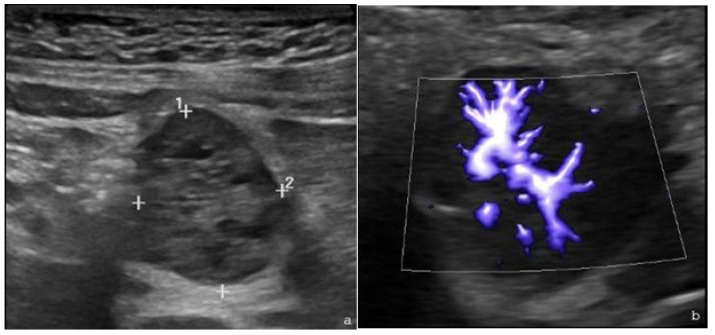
Abdominal US with echo–color Doppler in proband 1. (a) Abdominal B-mode US reveals a solid inhomogeneous hypoechoic formation with maximum dimensions 2.4 × 1.9 cm adjacent to the gallbladder and the left liver lobe. (b) Echo–color Doppler microvascular imaging (MVI) depicts the vascular hilum. The “+” symbols represent the ultrasound calipers used for lesion measurements. The accompanying numbers correspond to point identifiers and do not indicate lesion size.
